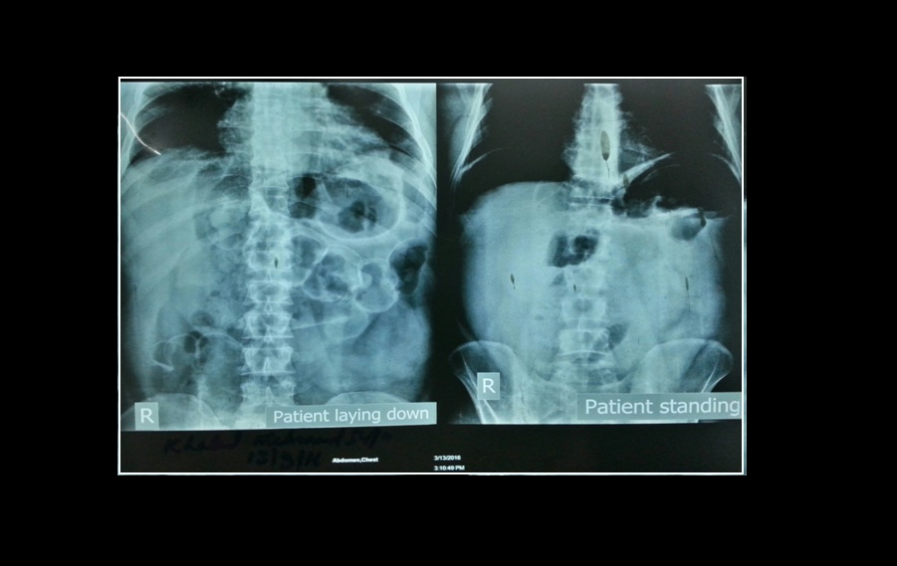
X-ray abdomen of the patient in supine (L) and standing (R) showing double wall appearance of the intestines (Rigler’s sign) with a clear liver edge and air under the diaphragm (‘Football’ sign). In the standing anteroposterior view, a bubble-like low density patch can be seen in the duodenal region showing a perforated site.
